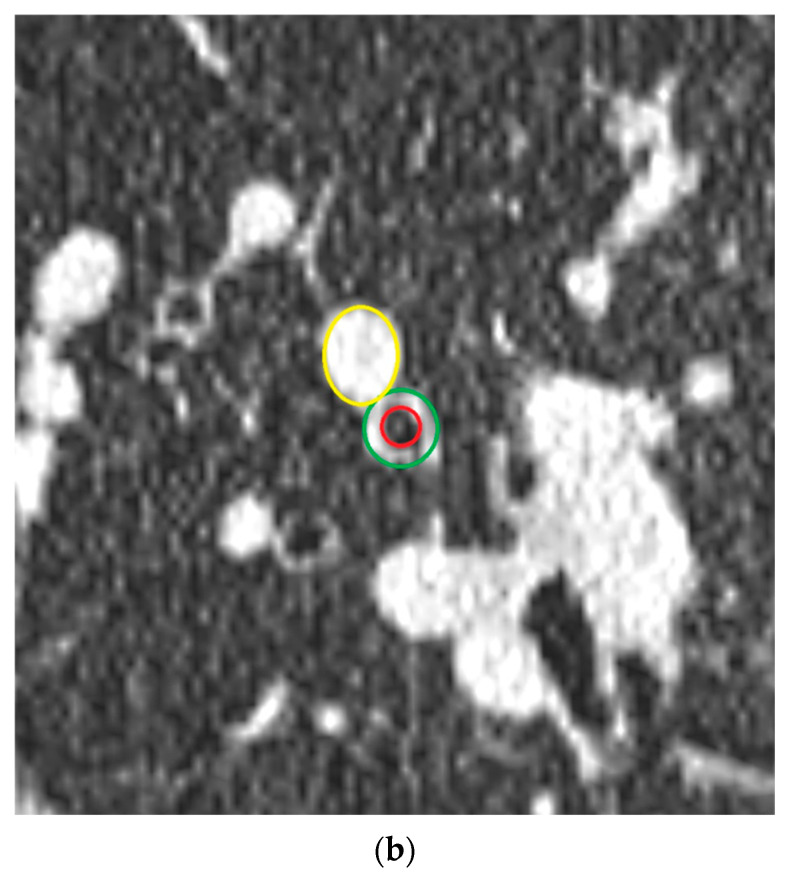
CT Airway measurement example. (a) is an example of segmented 3D volume rendered image of the bronchial tree generated from the CT data by SyngoVia image post processing software, with the segmental airway branches in various shades of green and blue. The red line signifies the bronchopulmonary segmental airway being measured in Figure 1b (left lower lobe lateral segment). (b) shows a corresponding multiplanar reformat image of the left lower lobe lateral segmental bronchus, double oblique to the centreline of the airway. Automated inner (red) and outer (green) airway area measurements are shown, along with measurement of the adjacent artery area (yellow).
